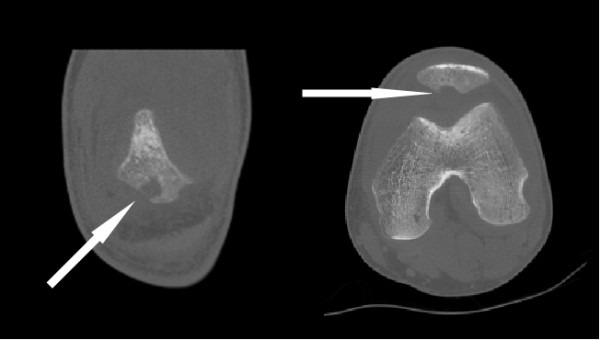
Coronal (left) and axial (right) images from a noncontrasted CT scan in bone algorithm (Width 2500, center 350; coronal 0.63 mm reconstruction; axial 1.25 mm slice) demonstrate more clearly, a focal area of osteolysis involving the inferior pole of the patella (white arrows).
